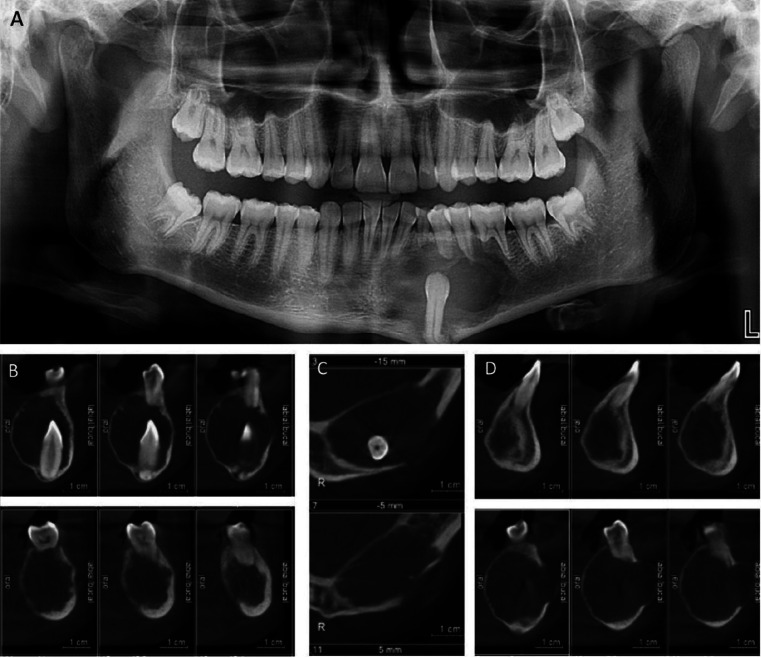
Panoramic X-ray and Cone beam computed tomography. A, Panoramic X-ray, extensive unilocular radiolucent lesion surrounding crown and root of tooth 3.3. It causes bulging of the basilar border, root resorption of teeth 7.3, 3.4, 3.5, 3.6 and dental displacement of tooth 3.1 and 3.2. Unerupted tooth 3.3 included in vertical position and displaced to basilar. B to D, Cone beam computed tomography, oval-shaped hypodense lesion causing marked root resorption of tooth 3.6 (B). Hypodense lesion causing expansion and thinning of vestibular and lingual tables, with perforation of the former (C). Oval-shaped hypodense lesion with peripheral hyperdense area located only vestibular to the lesion (D).
